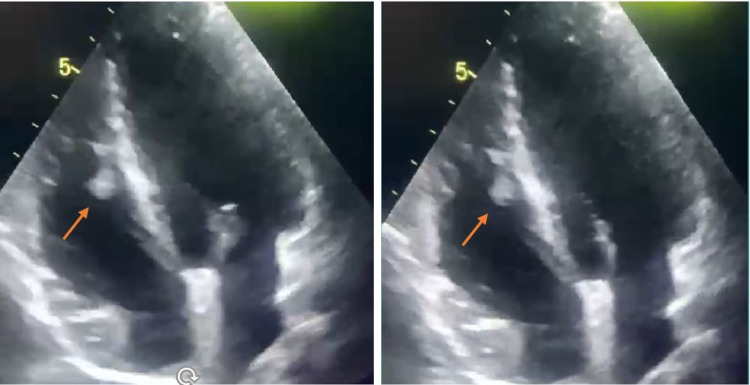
Echocardiography before anticoagulant initiation Transthoracic echocardiography showing mobile mural thrombus in the right ventricle.
